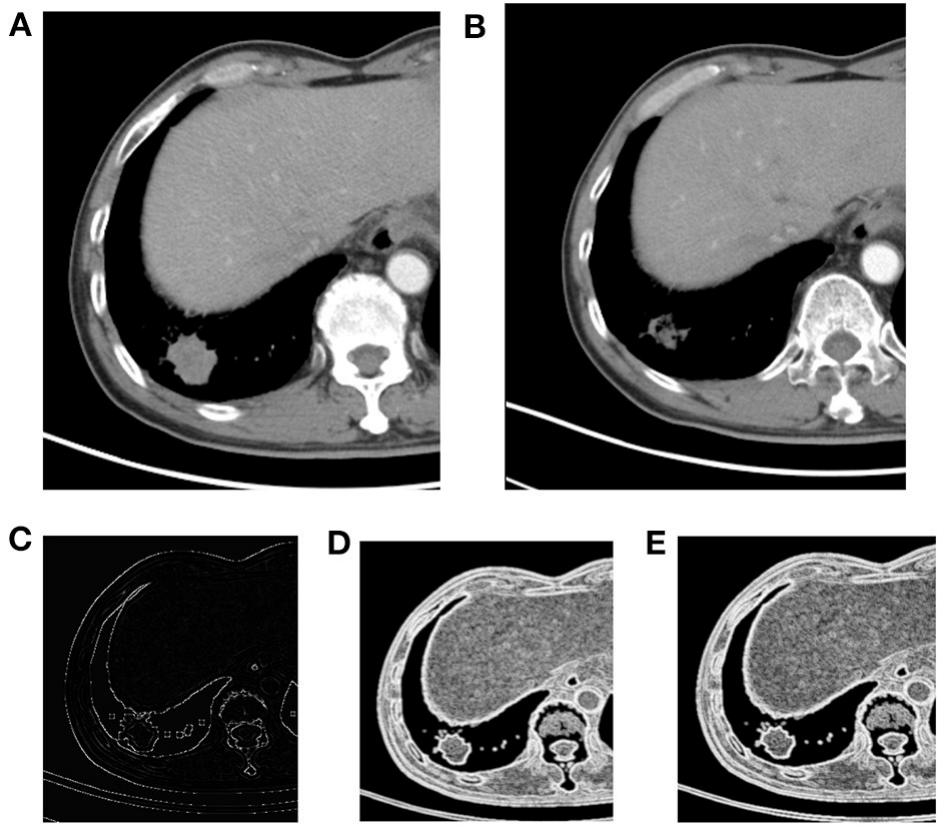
Right lower lobe nodule, NSCLC. (A) Pre-treatment contrast-enhanced; (B) contrast-enhanced CT 6 weeks later after treatment, the efficacy evaluation was partial response (PR); (C) kurtosis; (D) S(1,0) SumEntrp map; (E) S(2,2) SumEntrp map.
